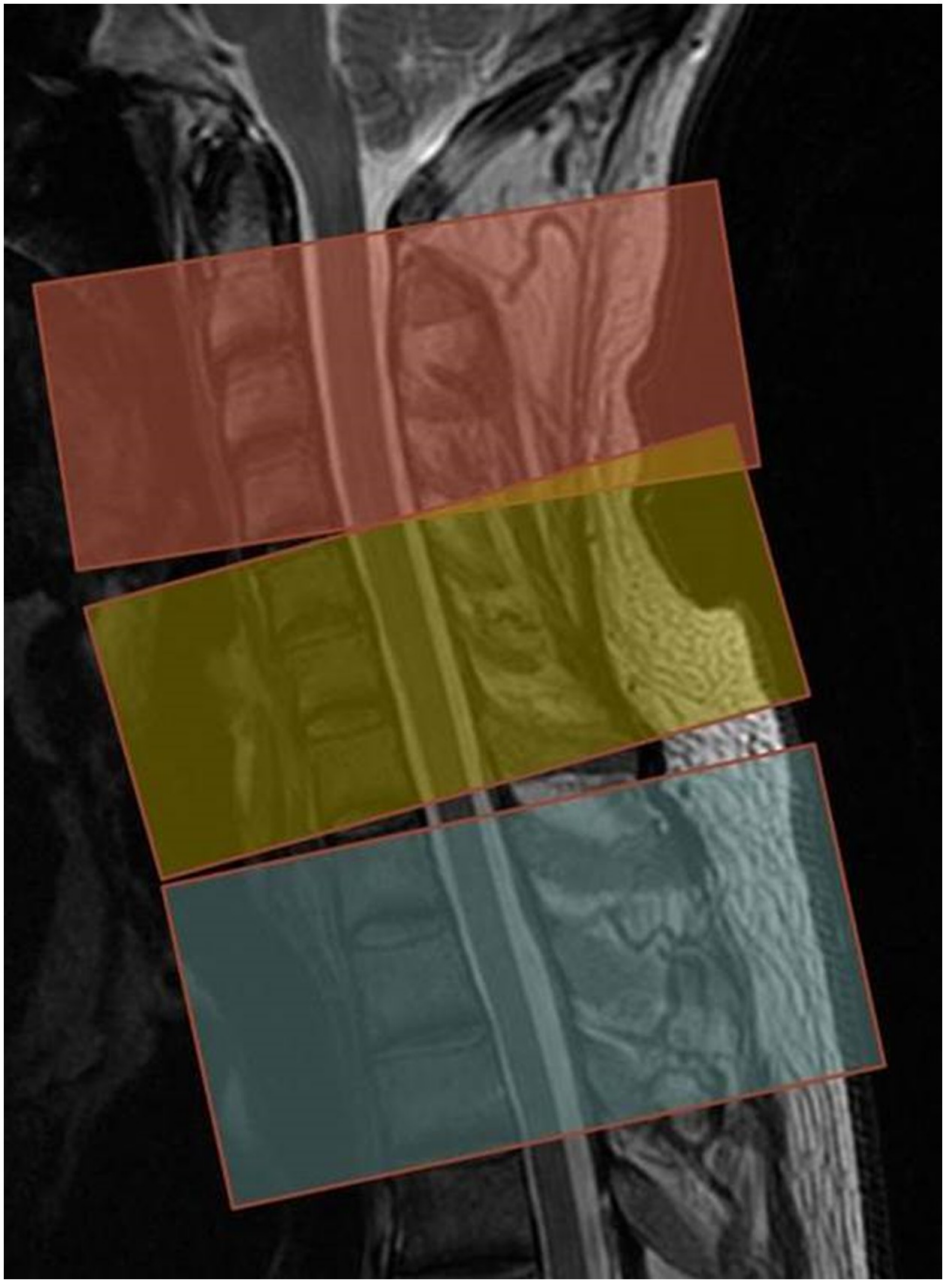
DTI planning. Sagittal T2-weighted image used for planning the axial sequences. The different-coloured blocks demonstrate the three levels of the axial DTI blocks.
